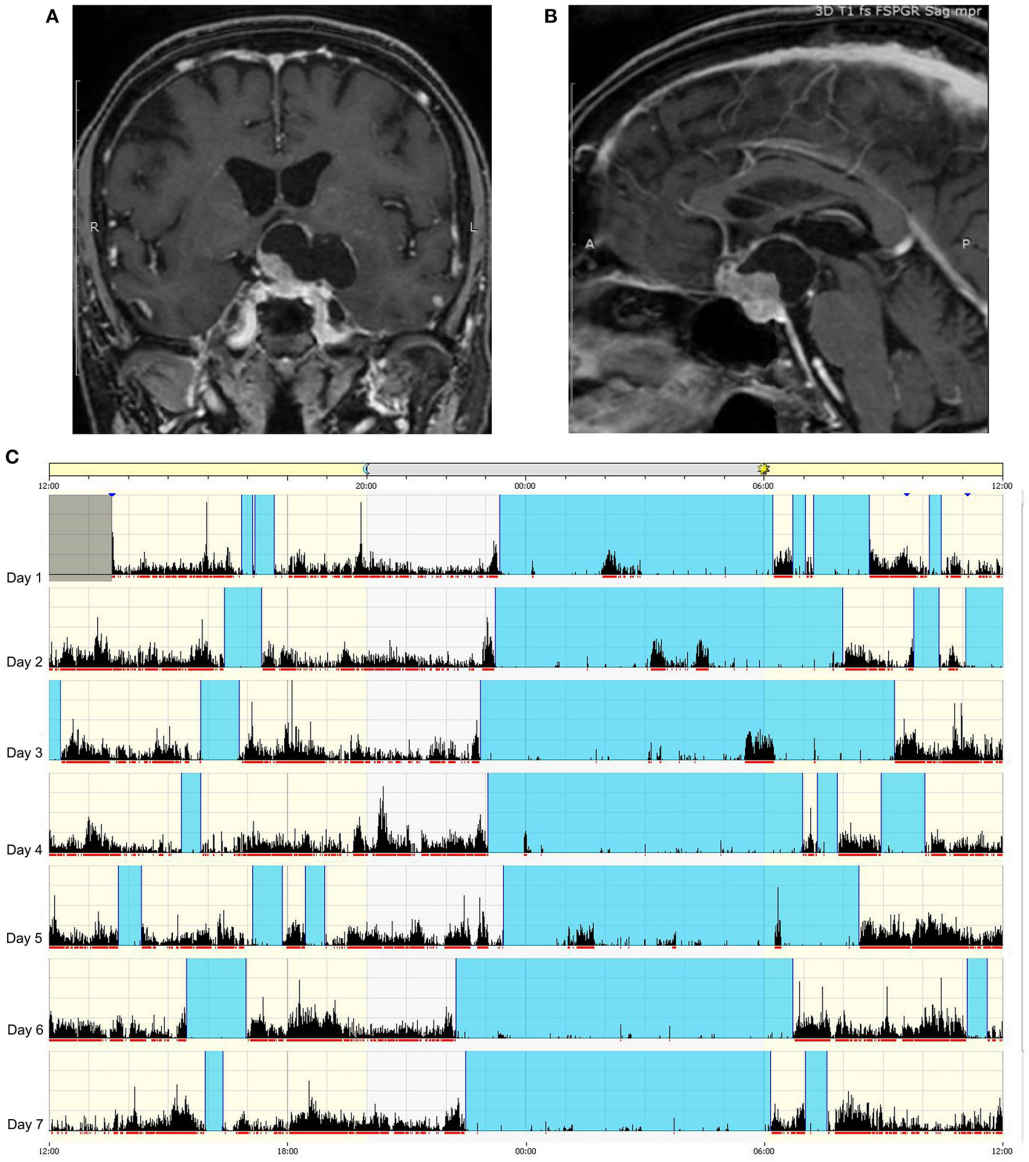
Example of circadian sleep-wake alteration evaluation by actigraphy. A 75-year-old female patient came to our observation because of headache and visual loss in the context of recent and rapidly worsening neurological symptoms consisting of insomnia, excessive daytime somnolence, cognitive impairment, reduced appetite, and weight loss. No poliurodyspia was present, and basal pituitary function and electrolytes were normal. Contrast-enhanced T1-weighted MRI revealed a mixed cystic and solid tumor consistent with suprasellar craniopharyngioma (A) with retrosellar extension (B). Sleep-wake patterns are displayed for individual days on actigraphy (C): vertical black bars and the red line under each day indicate movement, and the absence of black bars indicates supposed sleeping periods. The blue band designates the sleep period. The actigram shows frequent nighttime activity, severe insomnia, sleep fragmentation, and frequent short diurnal naps. The patient is currently awaiting surgery.
